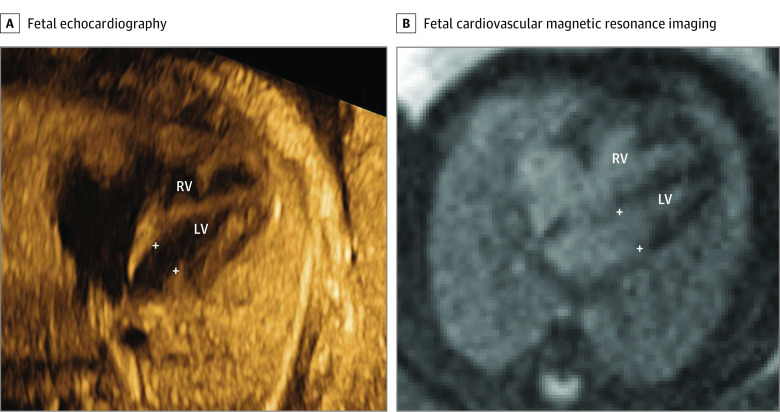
Measurement of Atrioventricular Valve Annulus for Assessment of Univentricular vs Biventricular Outcome A 4-chamber image by fetal echocardiography (A) showed an underdeveloped mitral valve annulus (5.6 mm; *z* score, −3.2) and a non–apex-forming left ventricle; however, the case was suspected to be underestimated or misdiagnosed due to suboptimal image projection. A 4-chamber image by fetal cardiovascular magnetic resonance (B) showed a normal-sized mitral valve annulus (9.4 mm; *z* score −0.3), 2 papillary muscles, and a narrow, almost apex-forming, left ventricle (LV) with normal function, suggesting biventricular outcome, as confirmed postpartum. White crosses indicate the mitral annulus. Contrast and brightness in the CMR image have been optimized for printing (case 13) (eTable in the Supplement). RV indicates right ventricle.
